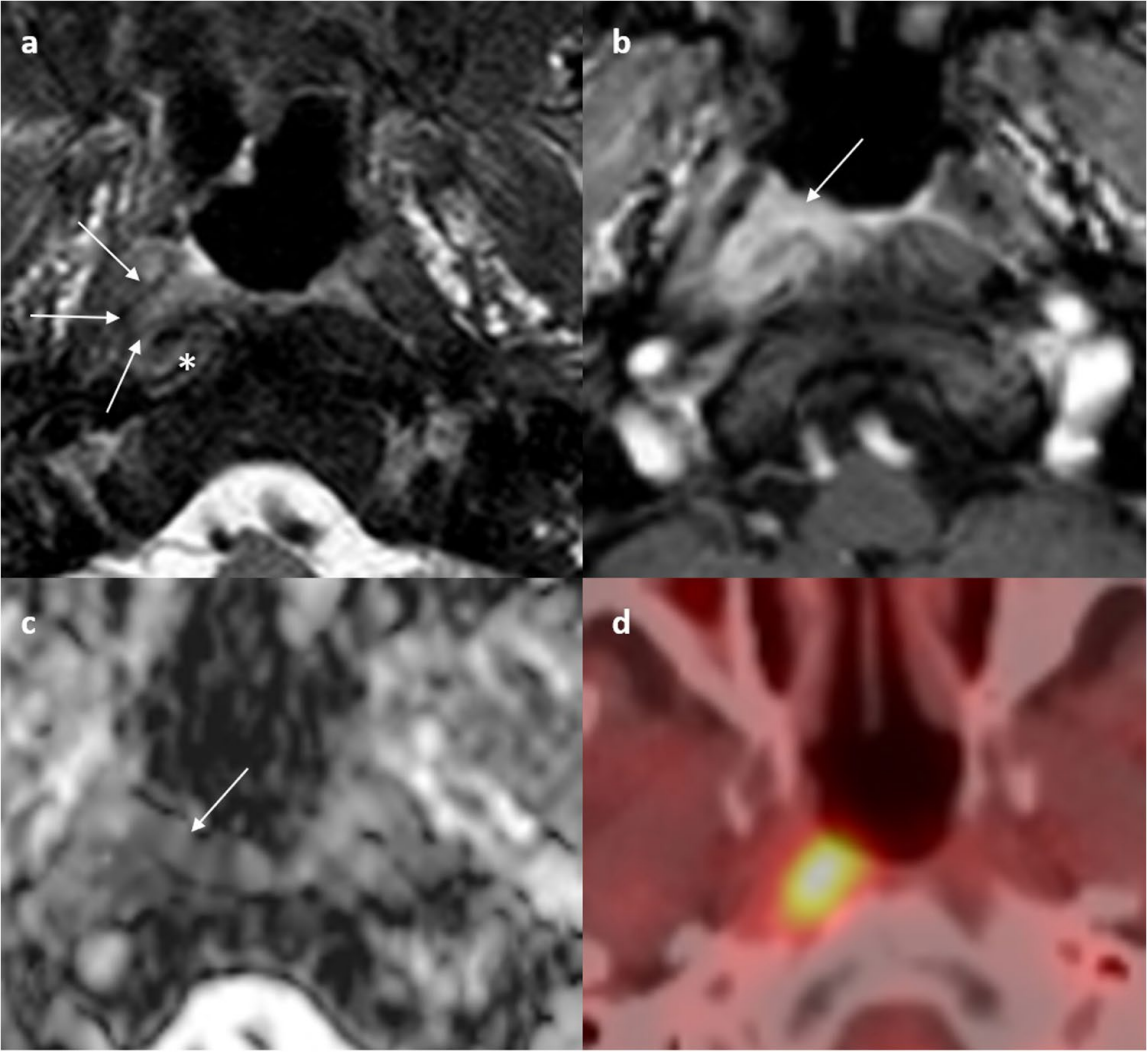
Typical signal characteristics of the recurrent nasopharyngeal tumor in a 72-year-old man with history of NPC (clinical stage T1N1M0) and radical radiotherapy completed in 2013. Axial T2-weighted image with fat-suppression shows an intermediate signal mass in the right nasopharynx causing focal expansion of the pharyngobasilar fascia which appears ill-defined (arrows). There is also extension of signal abnormality to the right prevertebral muscle (asterisk) (a). Axial T1-weighted image with fat-suppression shows the moderately enhancing mass in the right nasopharynx (arrow) with extension to right prevertebral muscle (arrow) (b). On the ADC map there is hypointense signal corresponding to the right nasopharyngeal mass (arrow) (c). Axial fusion FDG-PET/CT confirms the presence of a hypermetabolic mass in the right nasopharynx (d). Biopsy confirmed recurrent NPC
